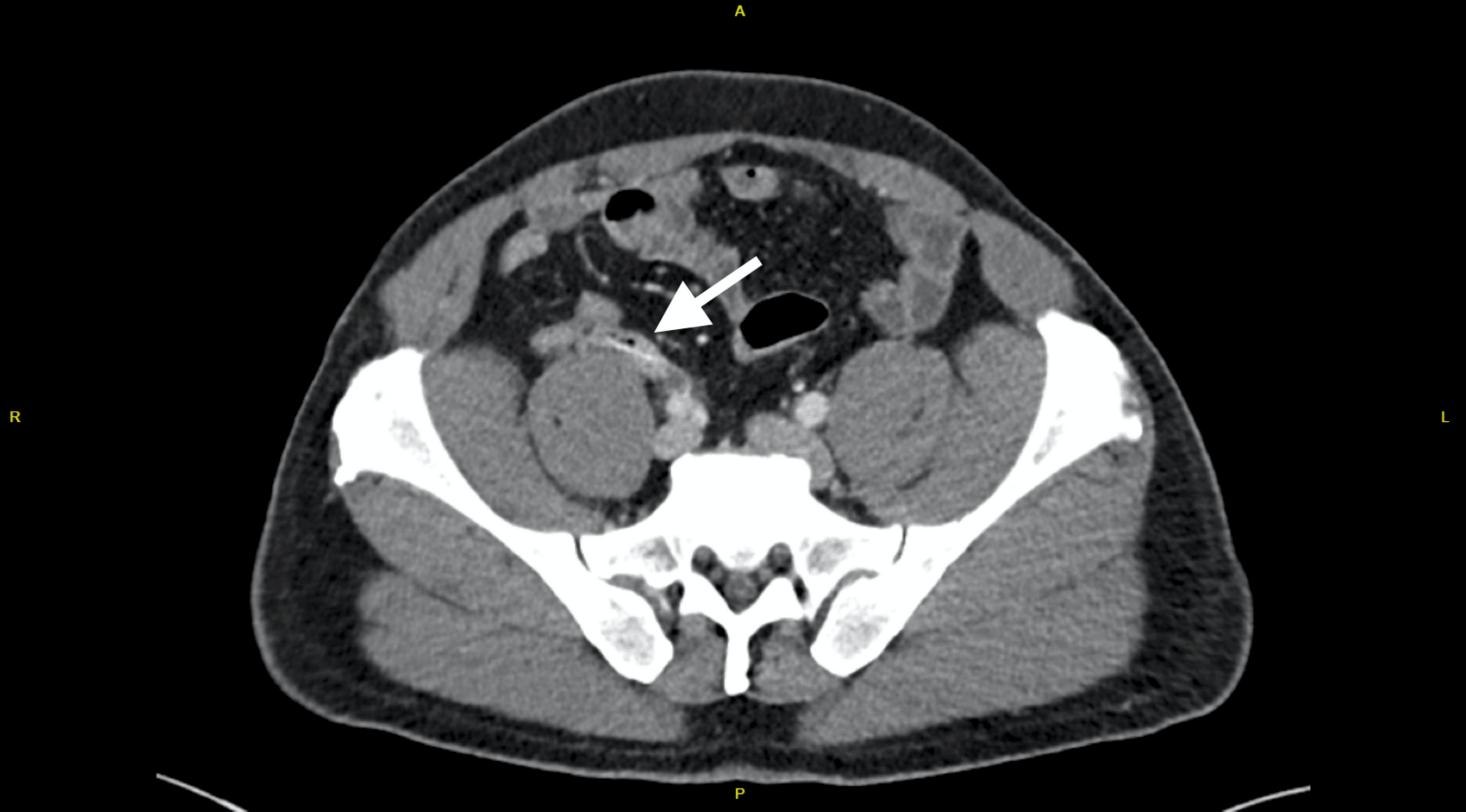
Axial contrast-enhanced CT scan of the abdomen revealed a dilated appendix with wall thickening indicative of acute appendicitis, along with a 2 cm linear hyperdense structure (white arrow).
